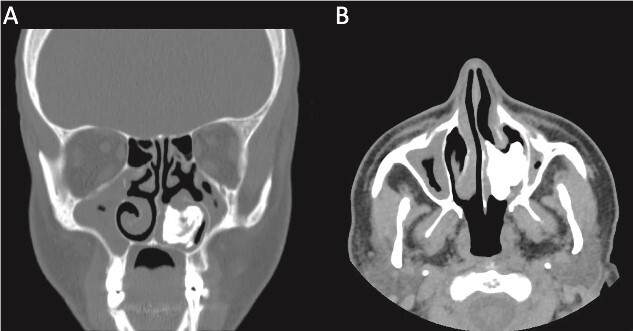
A CT scan with (A) coronal view and (B) axial view showing a well-defined expansile heterogeneous calcified/ossified lesion occupying the inferior aspect of the left nasal cavity.
